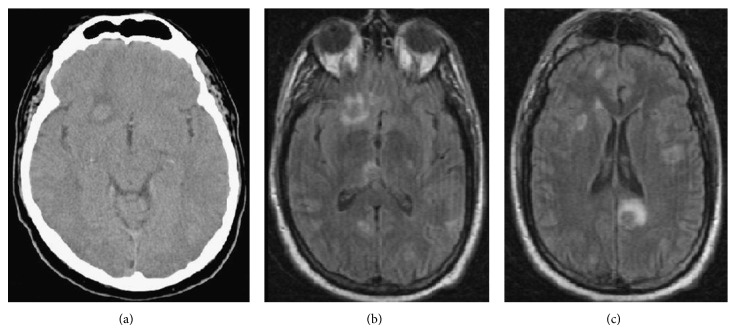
(a) Ct Brain 1.5 × 1.16 cm hemorrhagic right frontal lobe metastatic disease. (b, c) Postgadolinium MRI shows multiple ring enhancing metastatic lesions.
